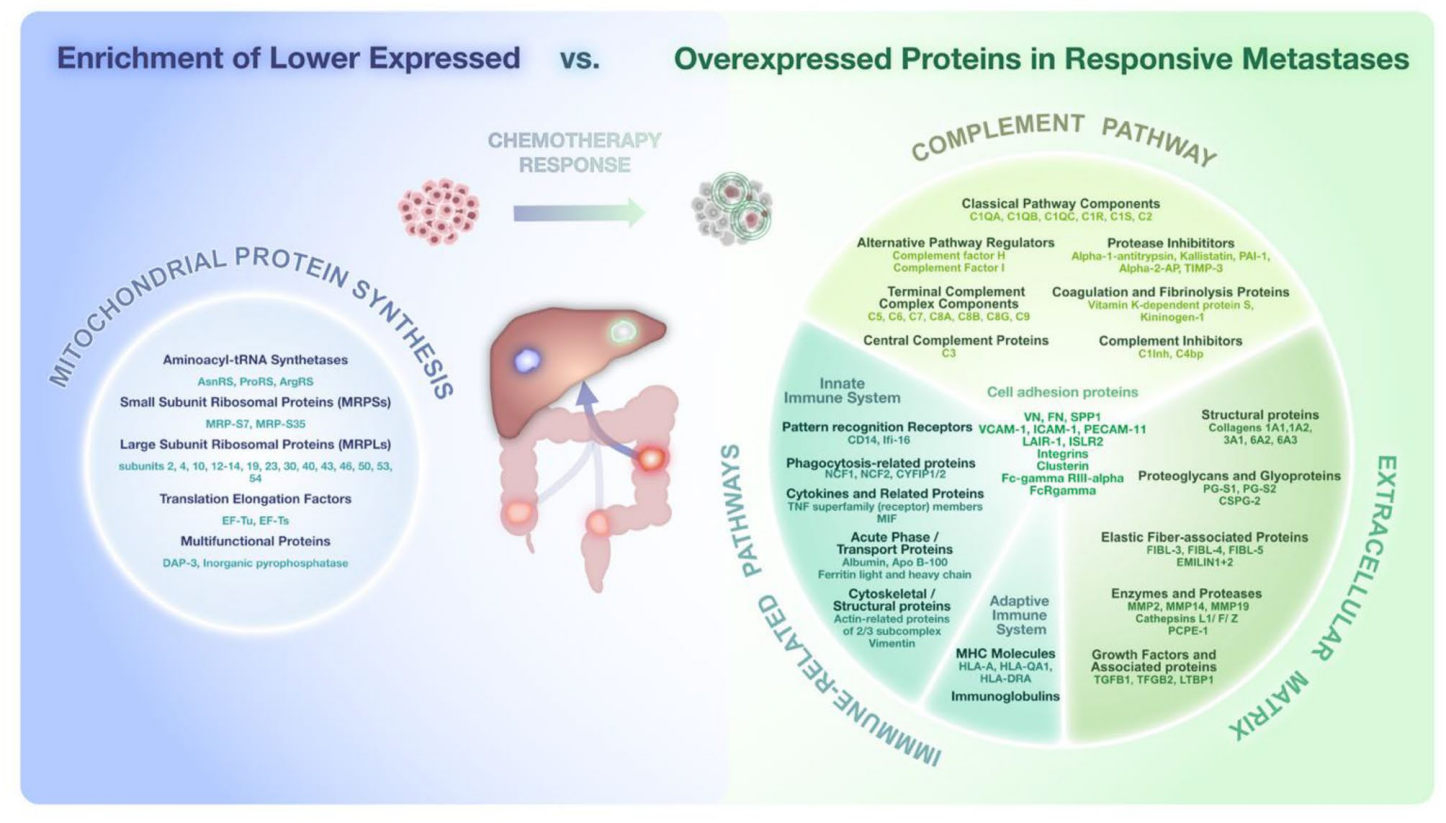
Proteomic enrichment of proteins with attenuated expression (left side) vs. proteins with elevated expression (right side) in CT-responsive metastases with MR
